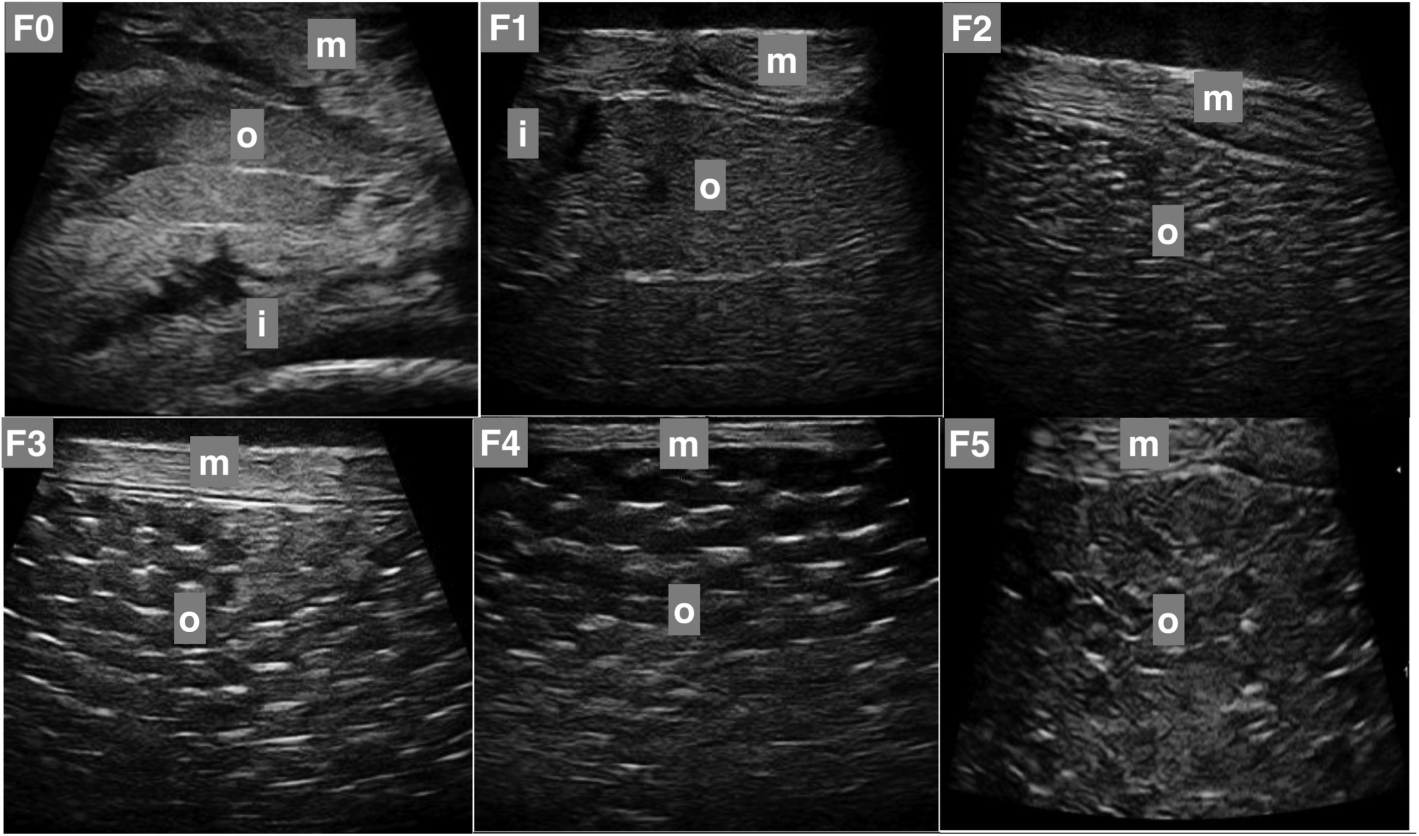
Ultrasound views of lumpfish ovaries. F0 to F5 are ultrasound gonadal stages from immature to spent ovaries. Apart from ovaries (o), intestines (i), and muscles (m) are also shown. The ultrasound gonadal stages are described in Table 1.
